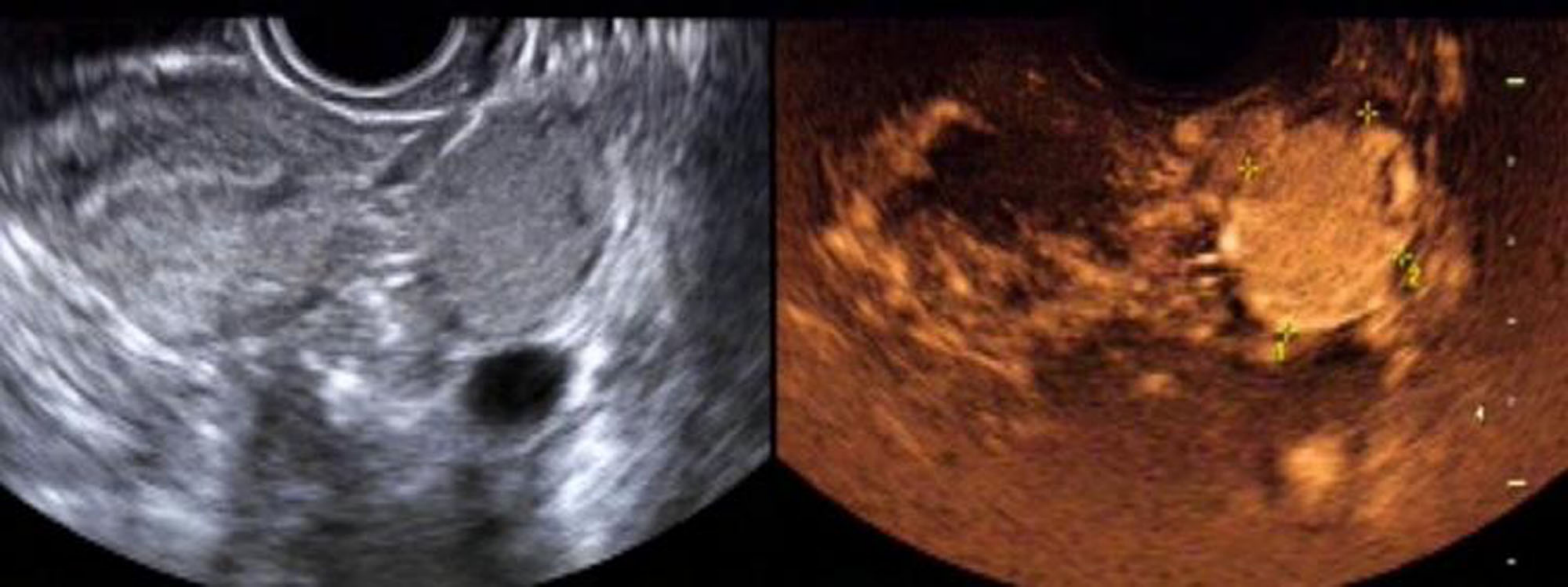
Female patient, 26 years old. Two-dimensional transvaginal ultrasound showed a round hypoecho in the left ovary, measuring about 3.5 cm × 2.4 cm × 3.1 cm, clear and regular in boundary, and uneven in internal echo. On CDFI, blood flow signals in the form of dots and strips were observed. According to contrast-enhanced ultrasound, the lesion in the left ovary was enhanced slightly earlier than that in the myometrium, and rapidly and integrally enhanced from multiple points in the center to the periphery, with the enhancement degree significantly higher than that in the myometrium. The lesion was cleared away from the center to the periphery, presenting as “fast forward and slow reverse”. The capsule echo appeared to be seen from the lesion in the early filling and late resolution.
